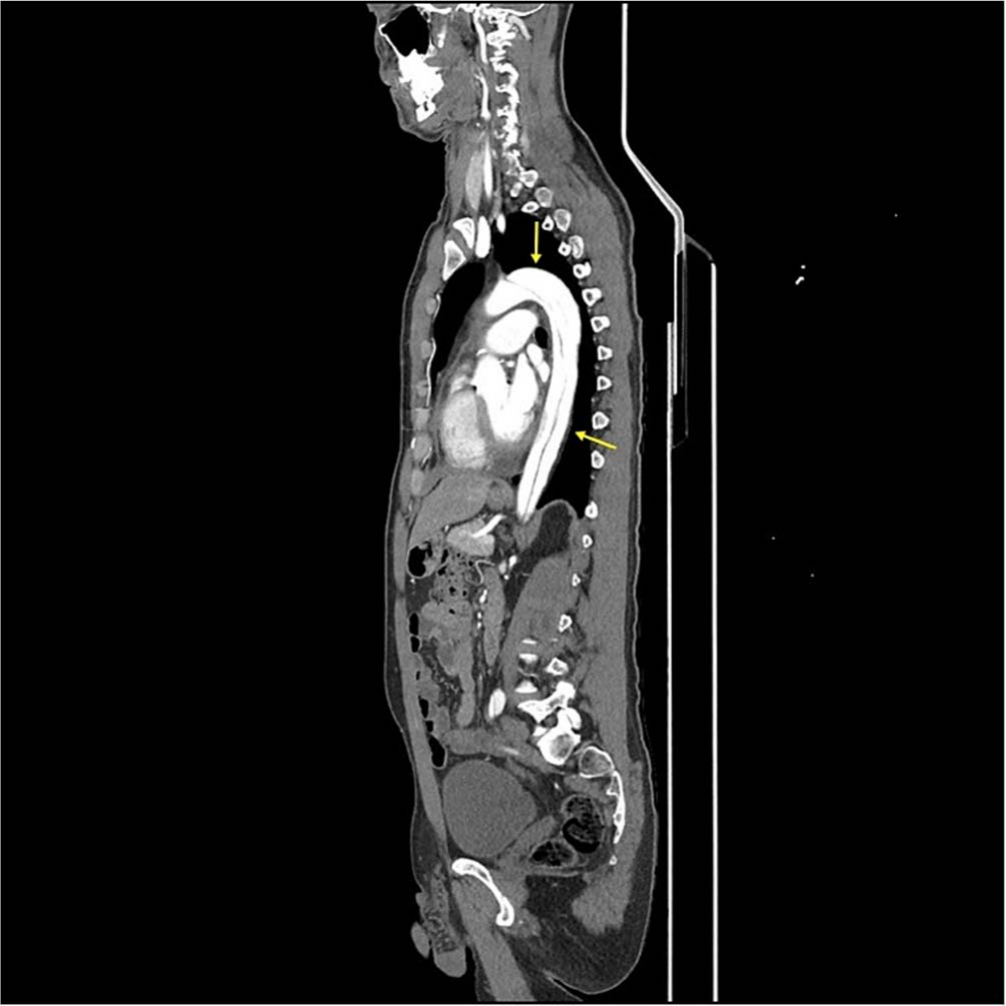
CT-scan pre-operatively showing Type A aortic dissection from the ascending aorta to the femoral arteries.
